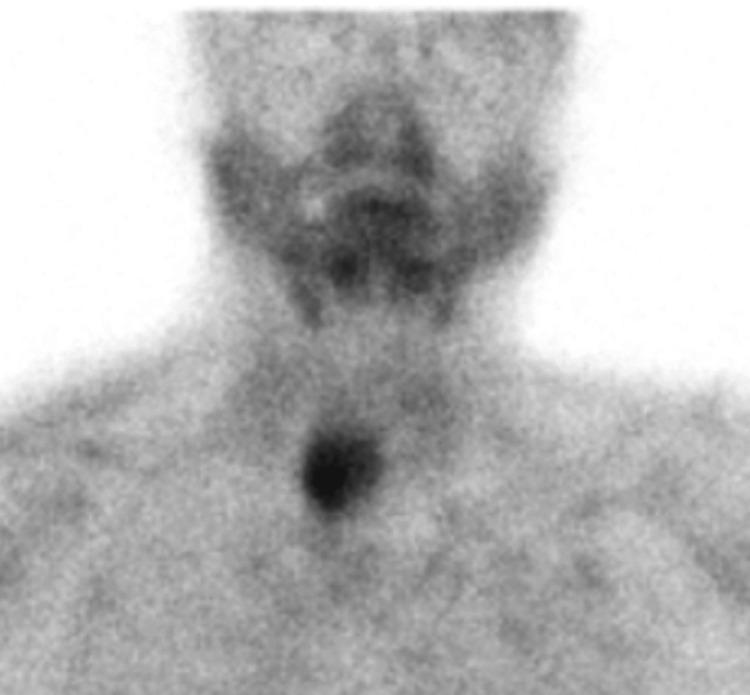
Sestamibi parathyroid scintigraphy showing an area of increased uptake adjacent to the right inferior thyroid pole.
